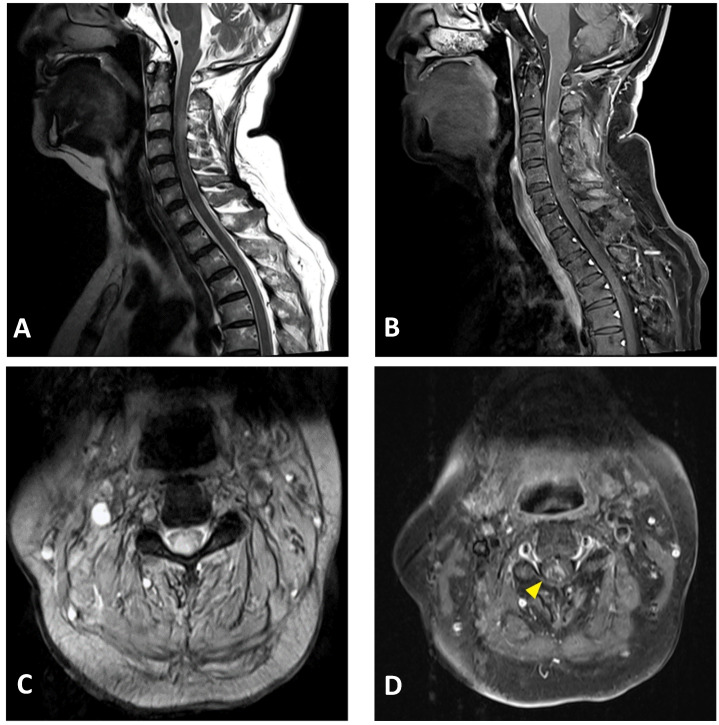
Cervical MRI images of a 76-year-old female with longitudinally extensive transverse myelitis: (A) sagittal T2-weighted image showing hyperintensity in the cervical cord at the C2–C5 levels; (B) sagittal T1-weighted image with contrast showing ring enhancement in the cervical cord at the C3 level; (C) axial T2-weighted image showing extensive hyperintensity in the cervical cord at the C3 level; (D) axial T1-weighted image with contrast showing right-sided enhancement in the cervical cord at the C3 level (arrowhead).
